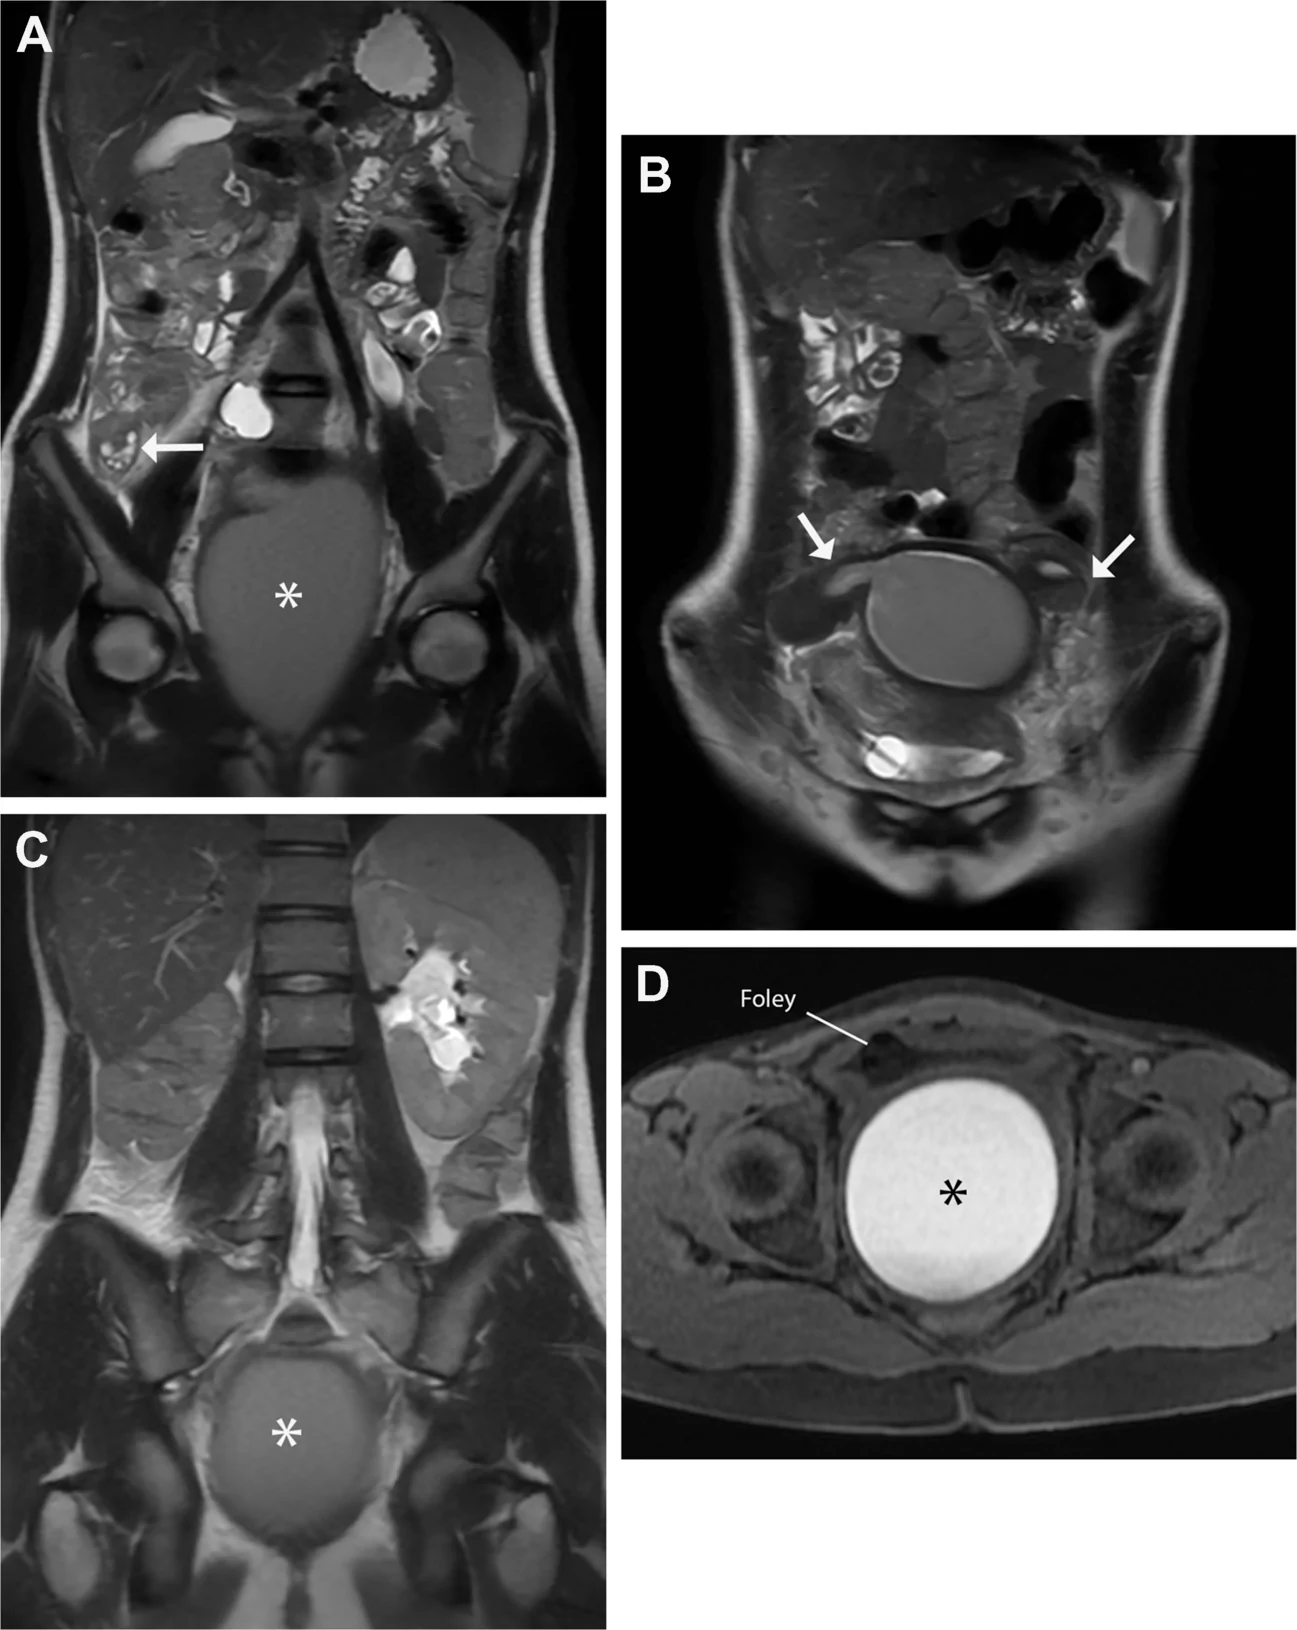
A 14-year-old girl with primary amenorrhea and obstructed hemivagina and ipsilateral renal agenesis (OHVIRA) on MRI. a-c Coronal T2-weighted images of the abdomen and pelvis and (d) axial T1-weighted fat-suppressed image of the lower pelvis show marked distention of the vagina (*asterisk*) by low T2-weighted and high T1-weighted blood products. Uterus is bicornuate, with laterally diverging uterine horns (*arrows*, b). Blood products are present in the right uterine horn. Normal ovaries are seen in the far lateral aspects of the pelvis bilaterally (*arrow* points to right ovary in a; left not shown). c Solitary left kidney with urinary tract dilatation is observed. d Distended vagina anteriorly displaces the urinary bladder, decompressed by a Foley catheter
